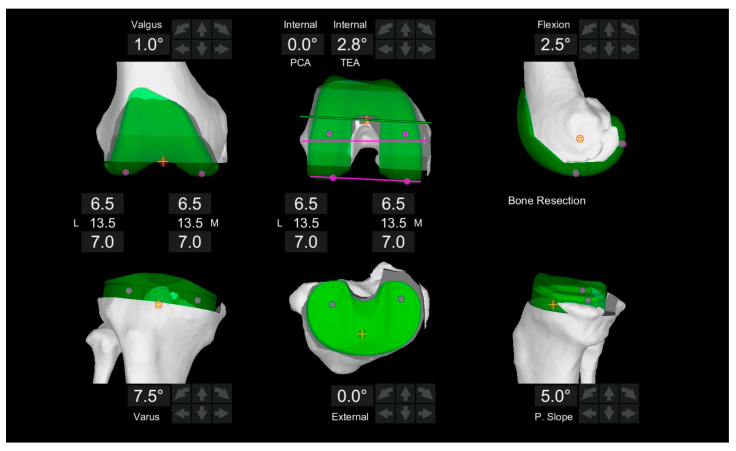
CT-based preoperative three-dimensional joint line orientation of the femur and tibia. Coronal alignment of the femoral and tibial components was planned by equalizing medial and lateral bone resections, aligning with the preoperative MPTA and LDFA. In this case, MPTA is 7.5° (varus), LDFA is −1.0° (valgus), and the posterior tibial slope (PTS) is 5.0°.
